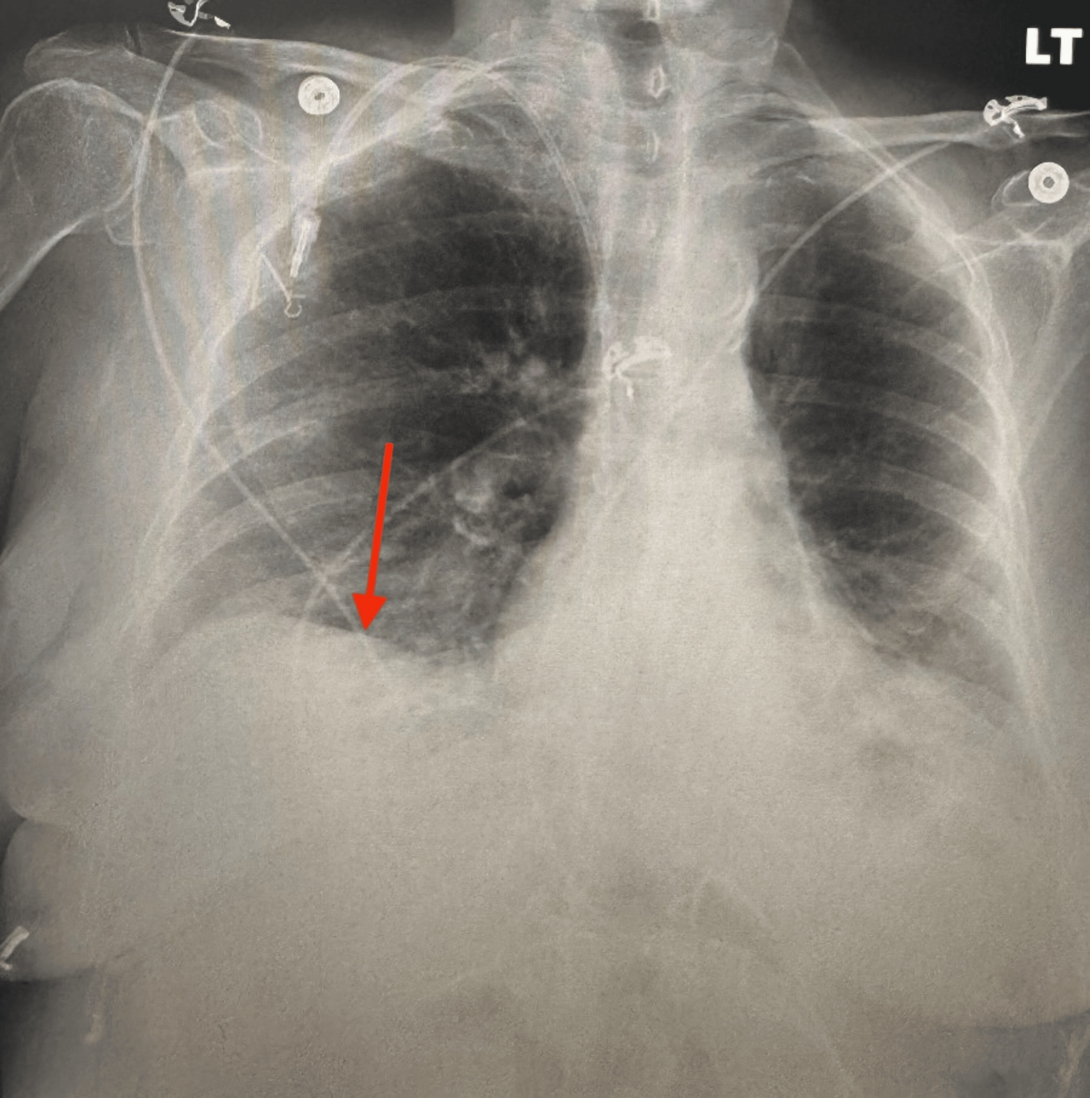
Chest X-ray on admission showing bilateral pleural effusions, more prominent on the right (red arrow)
